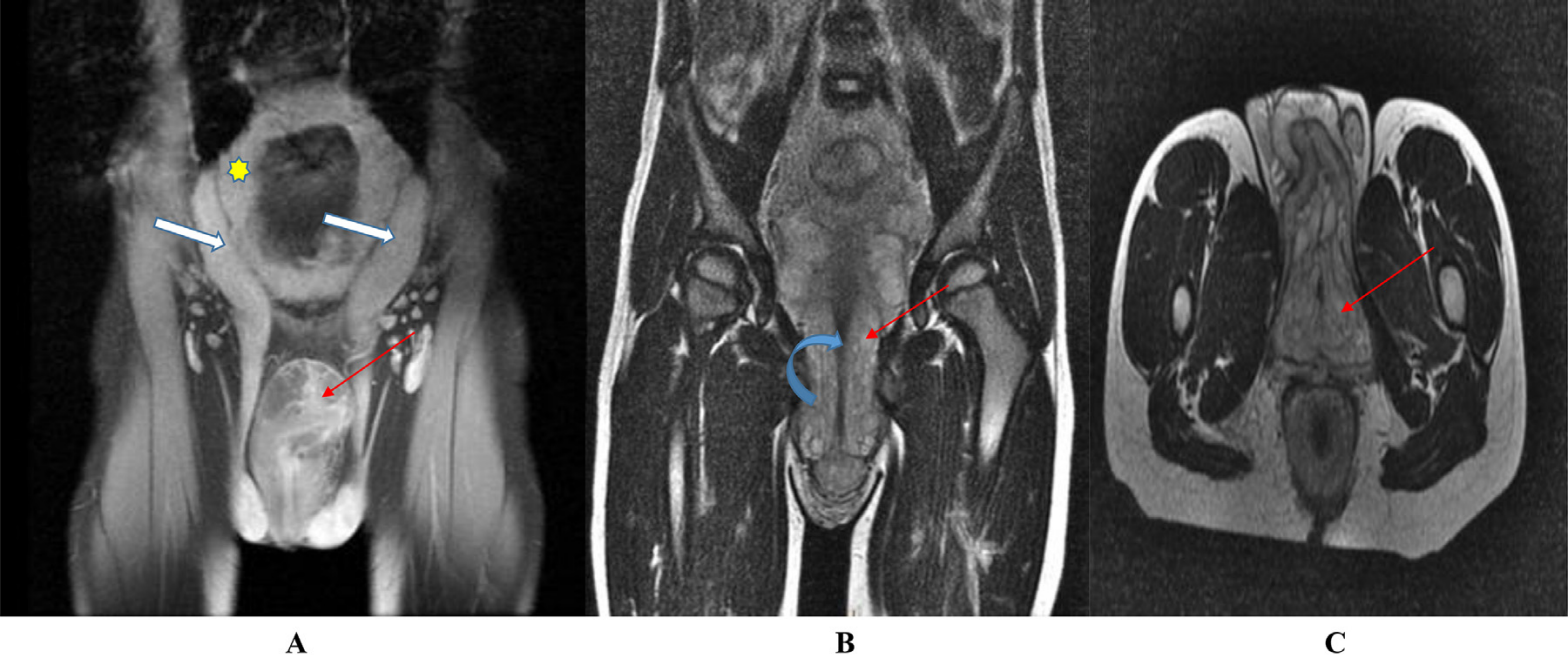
Pelvic MRI in the coronal T2 plane (A) and T1 (B), and in the axial T1 plane (C). • The spermatic cords (White arrows) (A) are enlarged, exhibiting diffuse and homogeneous infiltration with high T2 signal intensity. • Diffuse bladder wall thickening (Yellow star) (A). • Pelvic infiltration with extension towards the scrotal envelopes, infiltrating the membranous and spongy urethra (Blue arrow) and separating the corpora cavernosa, resulting in the thickened appearance of the penis (Red line) (B) (C).
